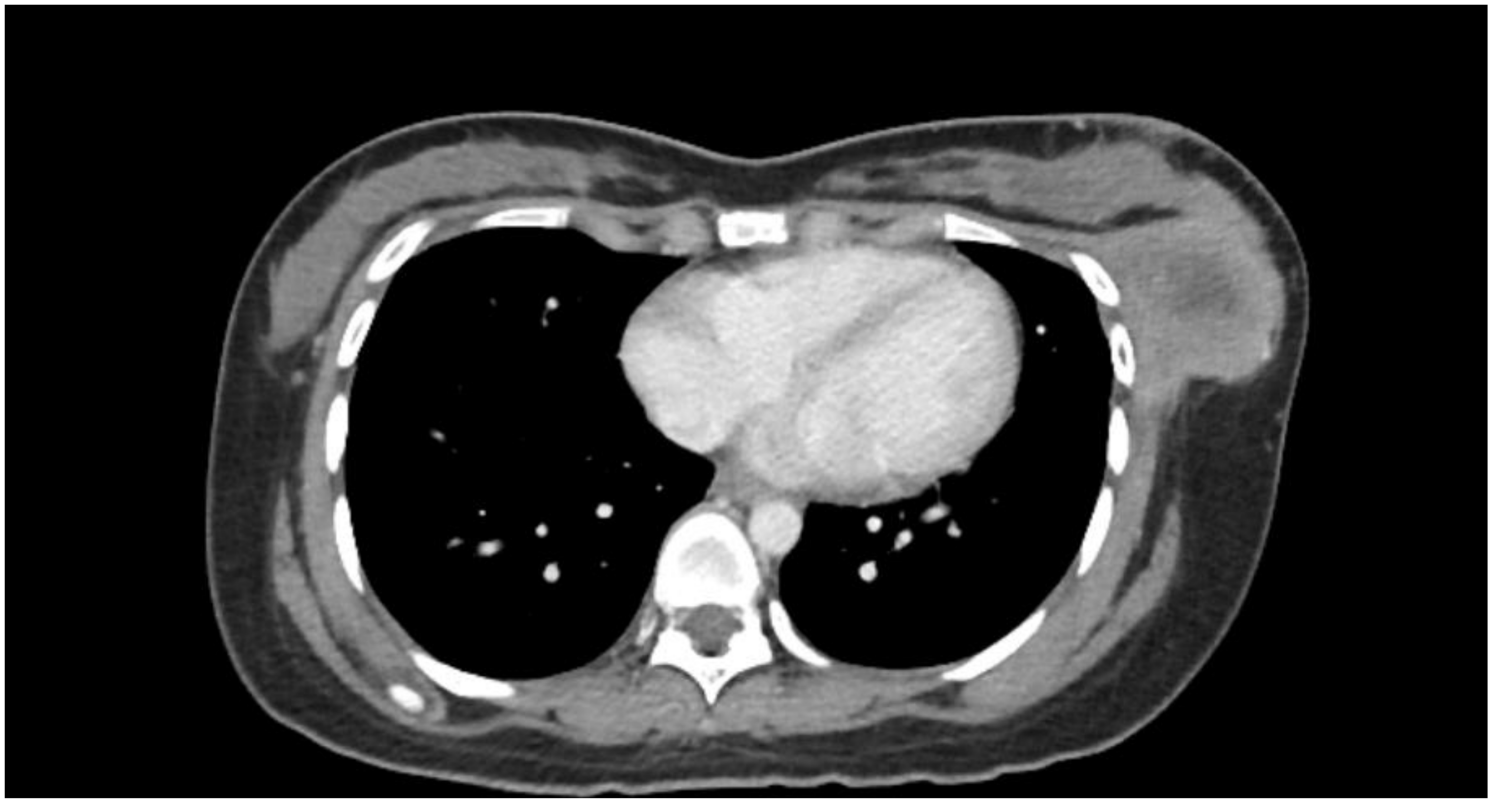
Computed tomography of the thorax showing a large tumour of the left breast.
